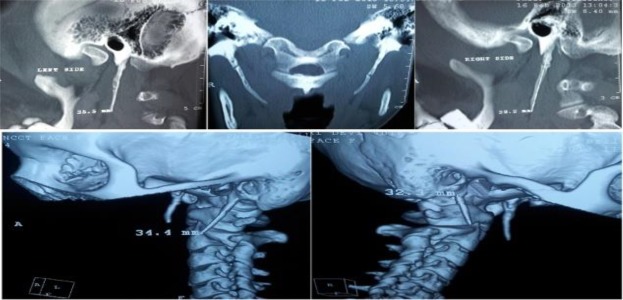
Various CT scan sections and with 3D reconstruction showing bilaterally elongated styloid processes
